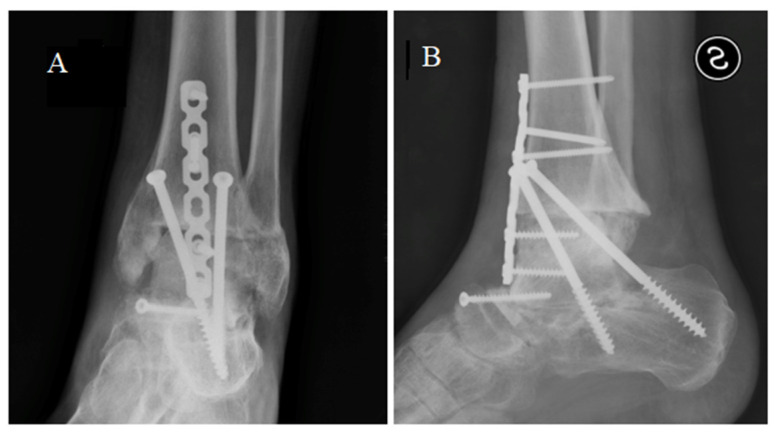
X-ray in anterior posterior (A) and latero-lateral (B) projections showing the tibio-calcaneal arthrodesis.
